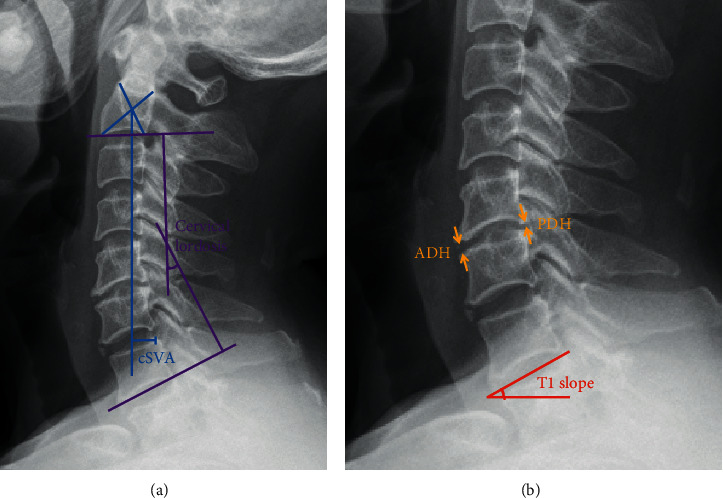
Radiographic measurement of the cervical sagittal alignment parameters. (a) cSVA: the distance between the plumb line from the center of C2 and the superior posterior corner of C7; CL: the angle between C2 (the inferior endplate) and C7 (the inferior endplate); (b) T1s: the angle between T1 (the superior endplate) and a horizontal line; ISH: average of anterior disc height (ADH) and posterior disc height (PDH). Abbreviations: cSVA, cervical sagittal vertical axis; CL, cervical lordosis; T1s, T1 slope; ISH, intervertebral space height; ADH, anterior disc height; and PDH, posterior disc height.
